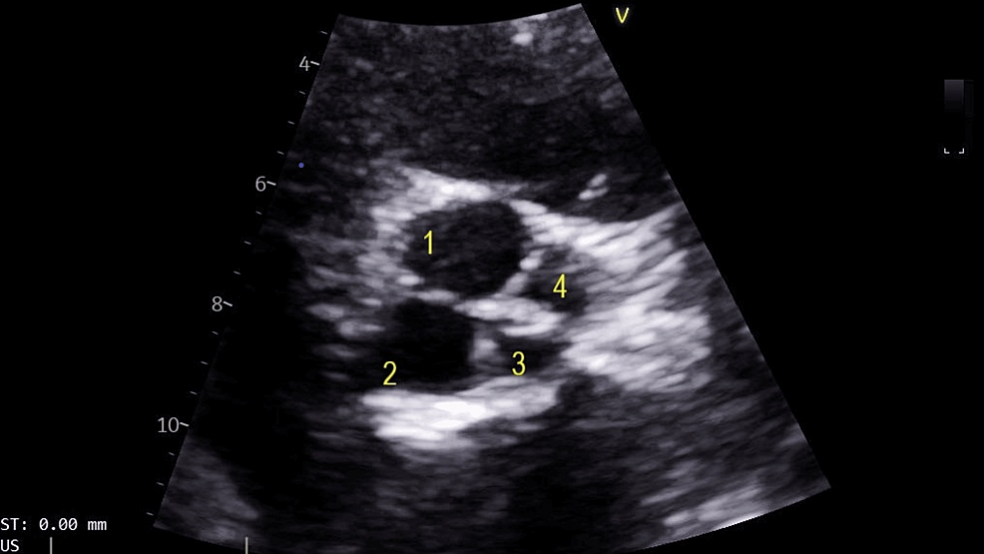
Transthoracic echocardiogram, diastolic view
